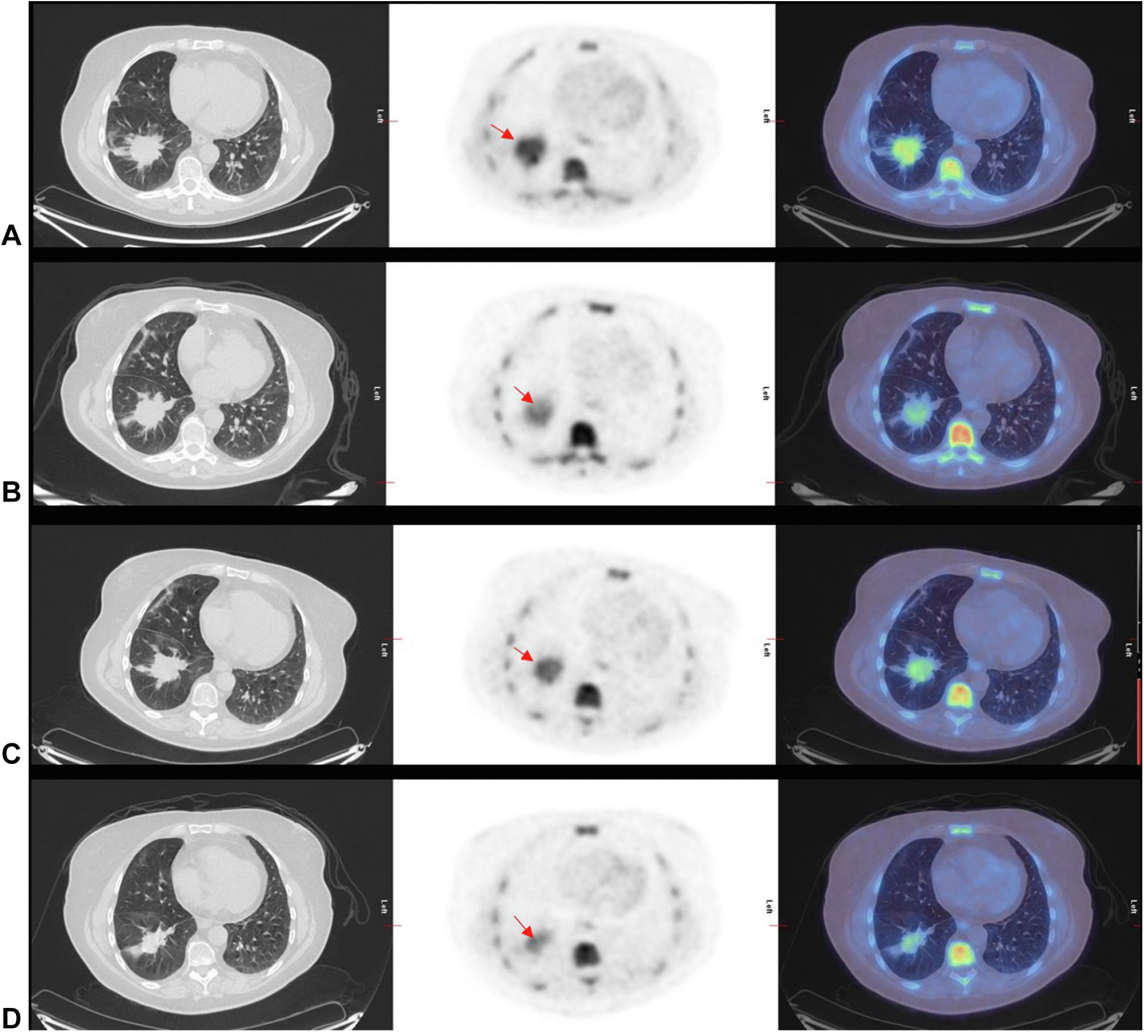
FLT PET-CT in NSCLC (case 11). (*A*) Baseline where primary lesion has SUVmax equals to 6.4 (red arrow). (*B*) Post–ADI-PEG20 at 24 hours, the SUVmax reduced to 4.8 (25% reduction hence PR). (*C*) Postcycle 1 of combined therapy, the SUVmax increased slightly to 5.4; however, at (*D*) end of treatment, the SUVmax decreased further to 4.1 (35% reduction from baseline) and hence PR was maintained. CT similarly had PR at end of treatment with a 33% reduction in size and StD at earlier time points. ADI-PEG20, pegargiminase; CT, computed tomography; EOT, end of treatment; FLT, 3'-deoxy-3'-fluorothymidine; MPM, malignant pleural mesothelioma; PET, positron emission tomography; PR, partial response; StD, stable disease; SUVmax, maximum standardized uptake value.
